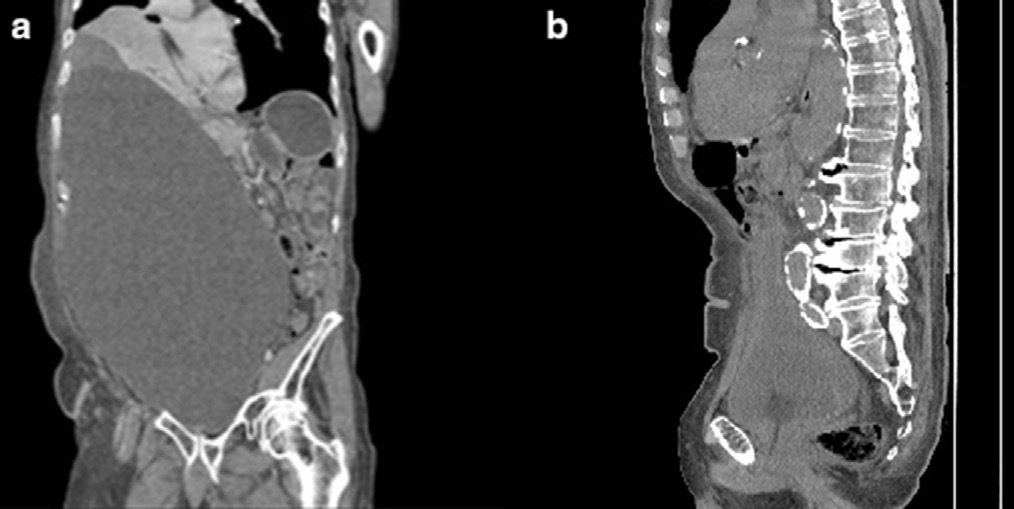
(a)Coronal CT image of abdomen and pelvis showed a better representation of the pelvic cystic mass. (b) Mid-sagittal view showing that the prostate is enlarged with a size of 4.3x 5.3 cm x 4.3 cm and a volume of 48.995 cm^3^.
